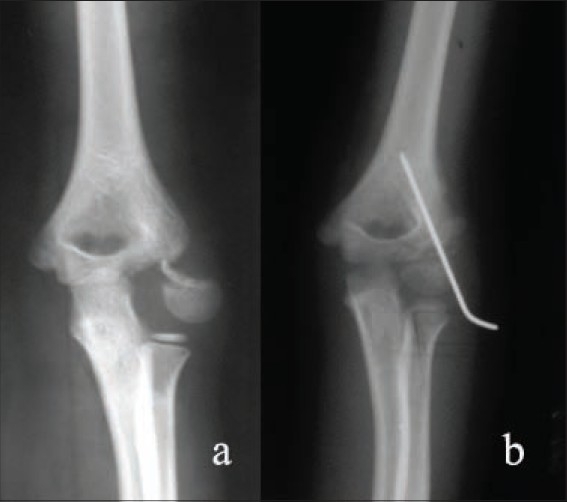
(a) Preoperative X-ray (anteroposterior view) of left elbow showing fracture lateral condyle of humerus, (b) Postoperative X-ray (anteroposterior view) of left elbow shows fracture fixed with bio-pin and additional K-wire because of stability concerns. The patient had full range of motion
